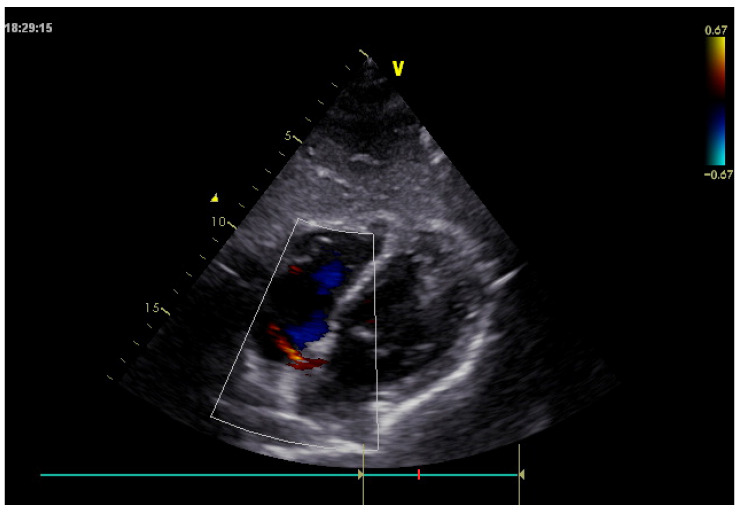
Transthoracic echocardiography showing absence of the Amplatzer device at the interatrial septum and evidence of a residual left-to-right shunt across the atrial septum.
